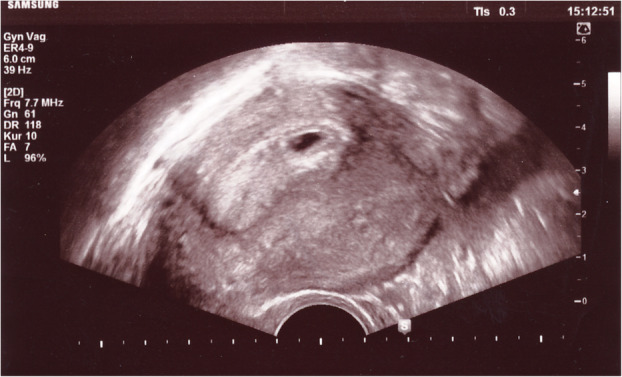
Spontaneous pregnancy of Patient W. 3 months after re-transplantation of ovarian tissue: ultrasound showing 3 week pregnancy
